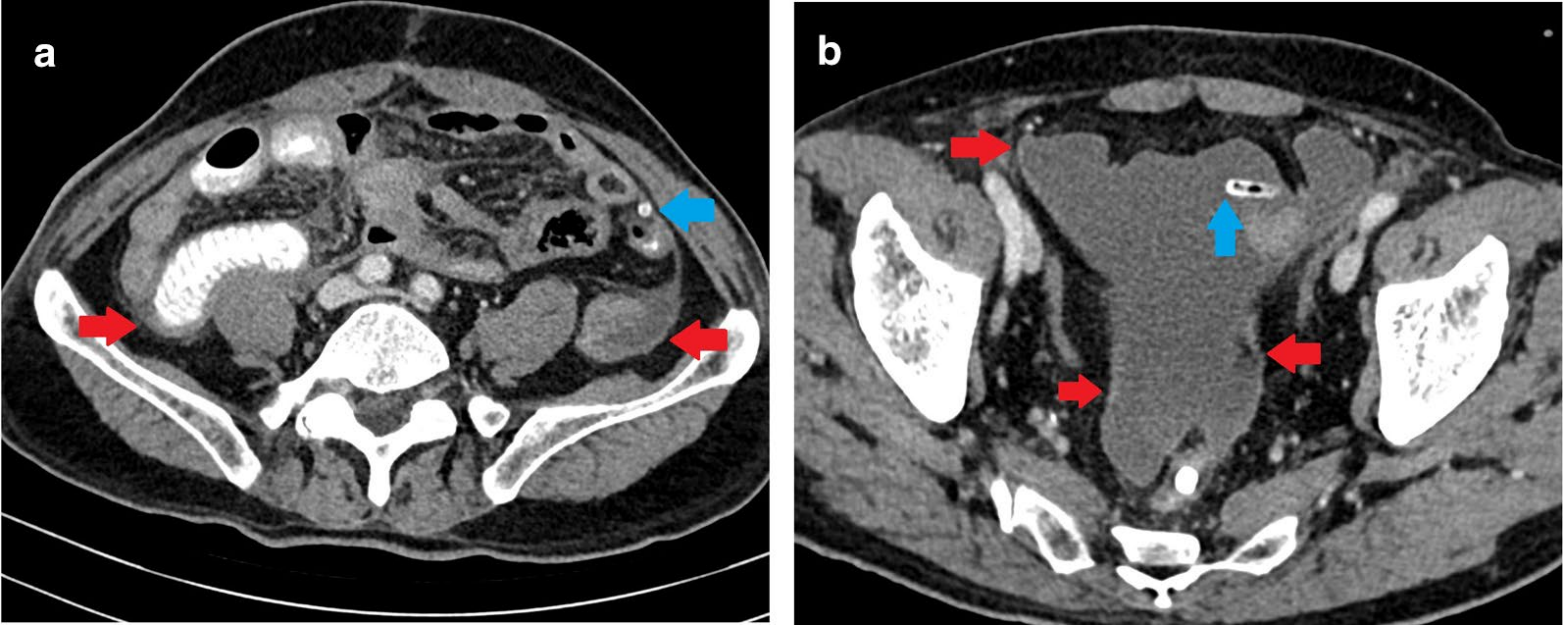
Premature closure bias causing an under-reading (type 4) error. A 52-year-old male patient with metastatic gastric cancer was brought to the emergency room due to abdominal pain, distension and discomfort. Abdominal CT showed thickened and enhanced peritoneal layers (red arrows a, b) and accompanying pelvic-free fluid/mesenteric congestion. The radiologist prematurely jumped to the conclusion of “peritoneal carcinomatosis” because of the cancer history and did not think about other reasons that could cause those imaging findings. In the following period, it was retrospectively understood that there was a displaced jejunostomy catheter (blue arrows a, b) into the peritoneal space. Due to premature closure bias, the radiologist did not pay enough attention to the rest of the examination and overlooked the displaced catheter. The patient was hospitalized and given long-term treatment. After removing the misplaced catheter and completing the appropriate treatment of peritonitis, induced by nutrients given through the jejunostomy, the patient’s complaints completely recovered
